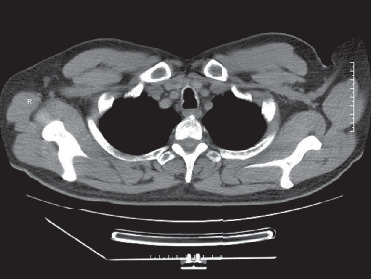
Case 3 lung CT: multiple ring calcifications in main bronchi walls.
